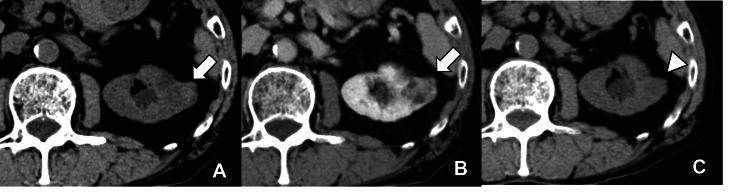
CT images of an 83-year-old man with clear cell RCC A: unenhanced image; B: enhanced image; C: unenhanced image 4 years before A, B: On axial UE-CT, a 2.2-cm heterogeneous hypodense mass is detected in the left kidney and enhanced heterogeneously (arrows). The mass protrudes from the outer segment of the left kidney. Pathologic examination confirmed that the mass was a 2.2-cm clear cell RCC. C: The renal mass was smaller and not more protruded from the outer segment of the left kidney (arrowhead). The mass was homogeneous and of low density on UE-CT, and therefore it was very difficult to be detected as malignancy 4 years before RCC: renal cell carcinoma; UE-CT: unenhanced computed tomography
